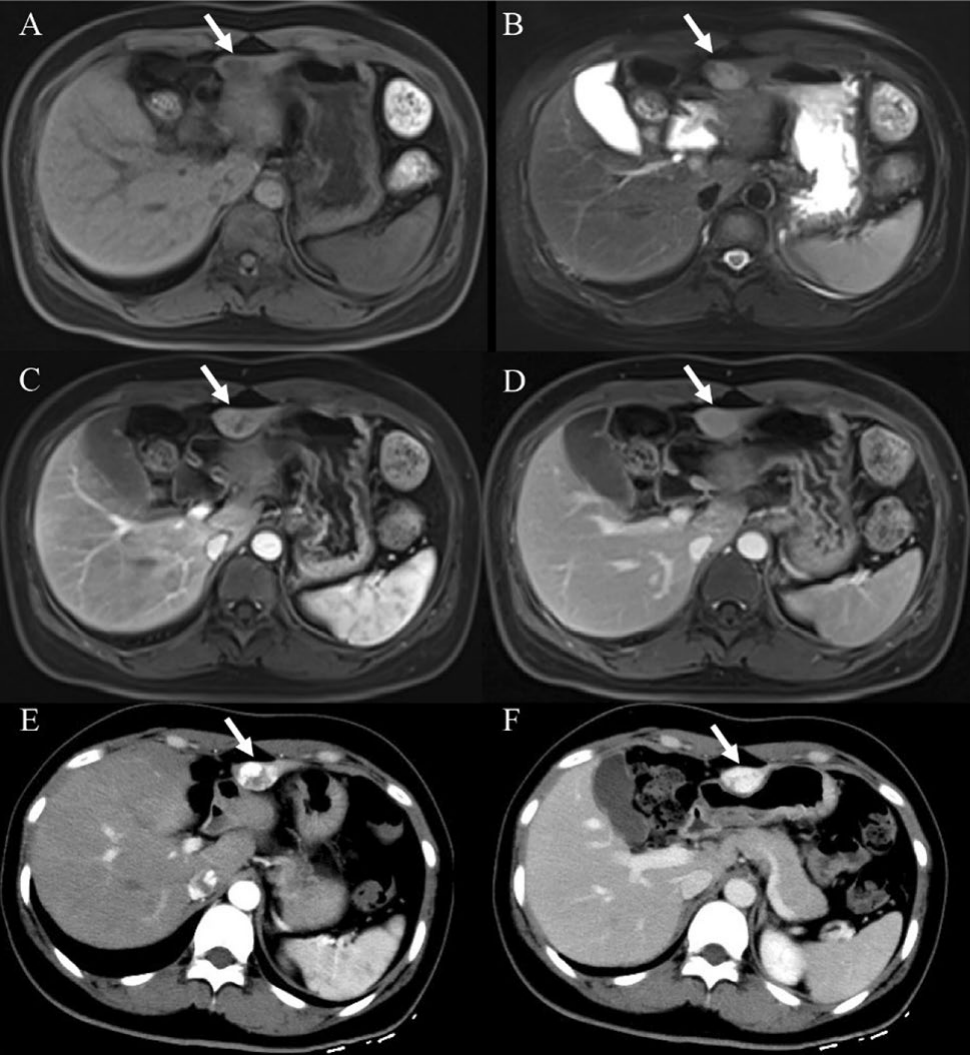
A case of sclerosing cavernous hemangioma mimicking hepatocellular carcinoma on MRI images (a 34-years-old female patient had chronic hepatitis B for more than 10 years). The mass was located in segment 3. It showed slightly hypointense on T_1_-weighted fat-suppressed image (A), slightly hyperintense on T_2_-weighted fat-suppressed image (B), heterogeneous enhancement in the arterial phase (C), and a subtle low-signal intensity with capsular enhancement in the delayed phase (D). Based on MRI findings, well-differentiated hepatocellular carcinoma was suspected. CT was further performed to rule out hemangioma. Enhanced CT demonstrated a typical nodular enhancement in the arterial phase (E) and continued filling in during the portal venous phase (F) (arrows)
